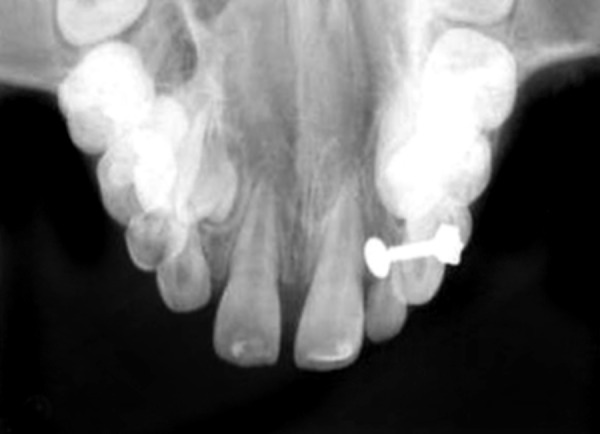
Occlusal radiograph showing bilateral congenital absence of lateral incisors
